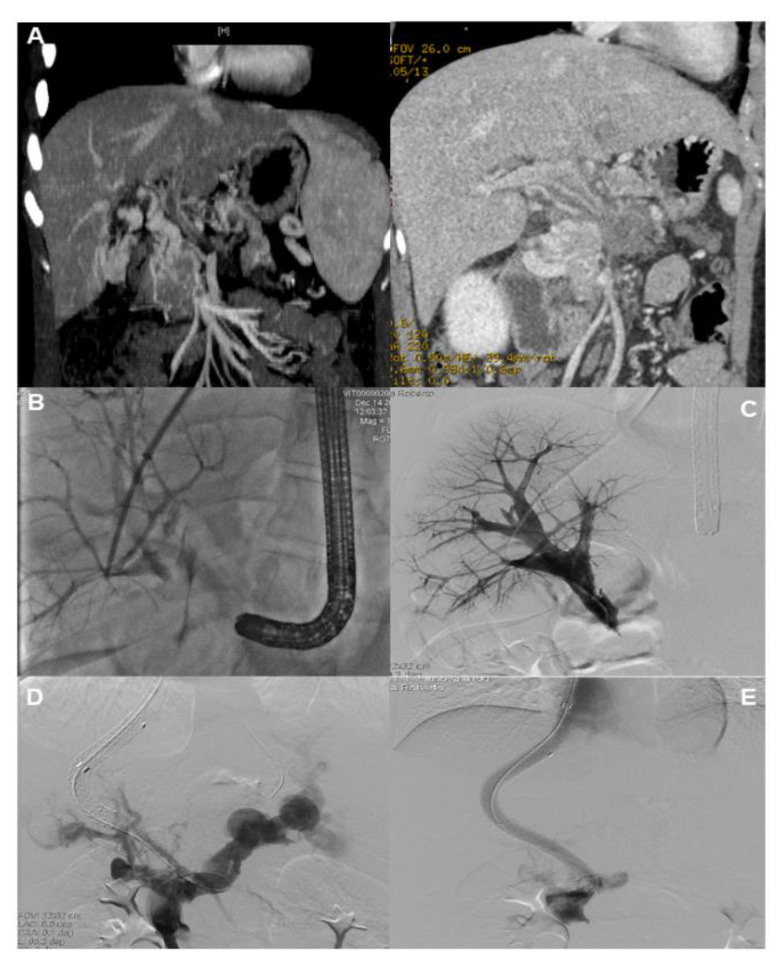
Example of a patient with noncirrhotic nonmalignant portal vein cavernoma secondary to umbilical vein catheterization in the neo-natal period. The patient was admitted for uncontrolled upper gastrointestinal bleeding from a duodenal varix, which could not be managed endoscopically; thus, the creation of an emergency TIPS was attempted. (A) Abdominal CT scan performed before the bleeding episode, showing the portal cavernoma. (B) The Colapinto needle was advanced from the right hepatic vein into a portal vein radicle using real-time sonographic and fluoroscopic guidance. (C) Injection of contrast shows tracking along the portal vein remnant, with stasis at the caudal aspect due to the absence of communication between the remnant and the spleno-mesenteric confluence. (D) A hydrophilic wire was successfully introduced through the remnant and into the spleno-mesenteric confluence. (E) This was substituted with a stiff wire, over which two stents were deployed in series.
